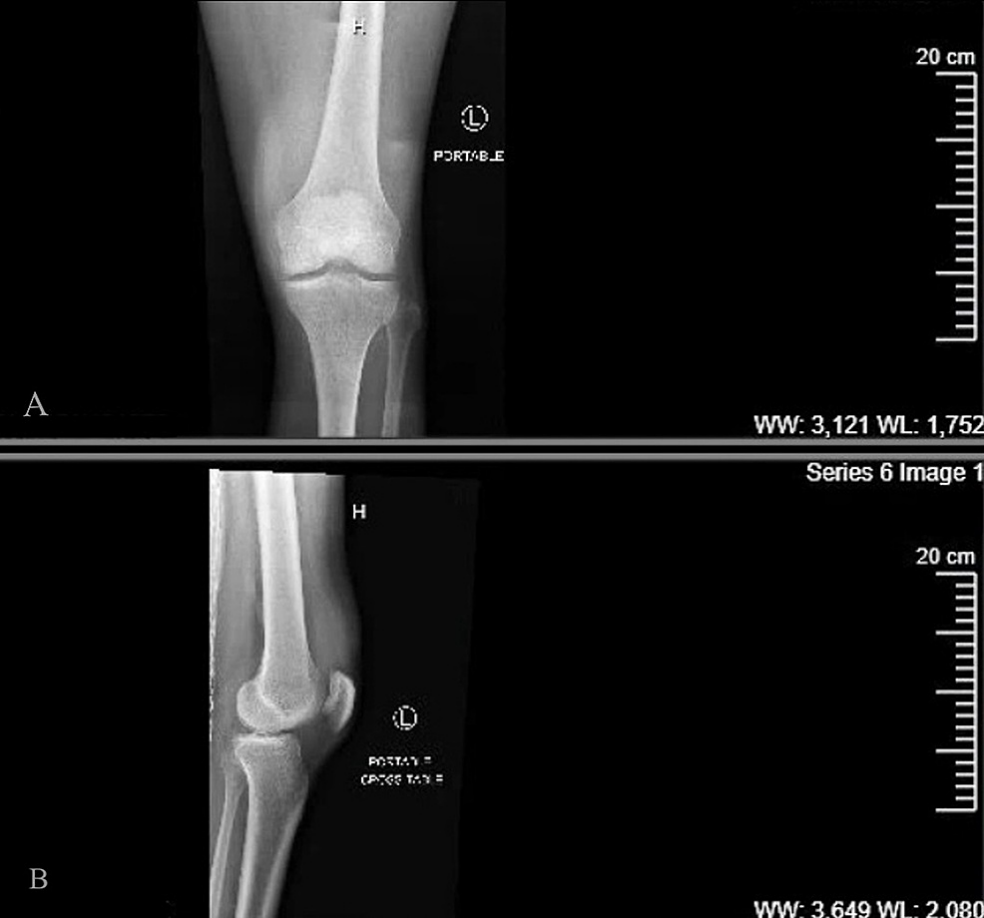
X-ray of the left knee. (A) Anteroposterior view. (B) Lateral view.
